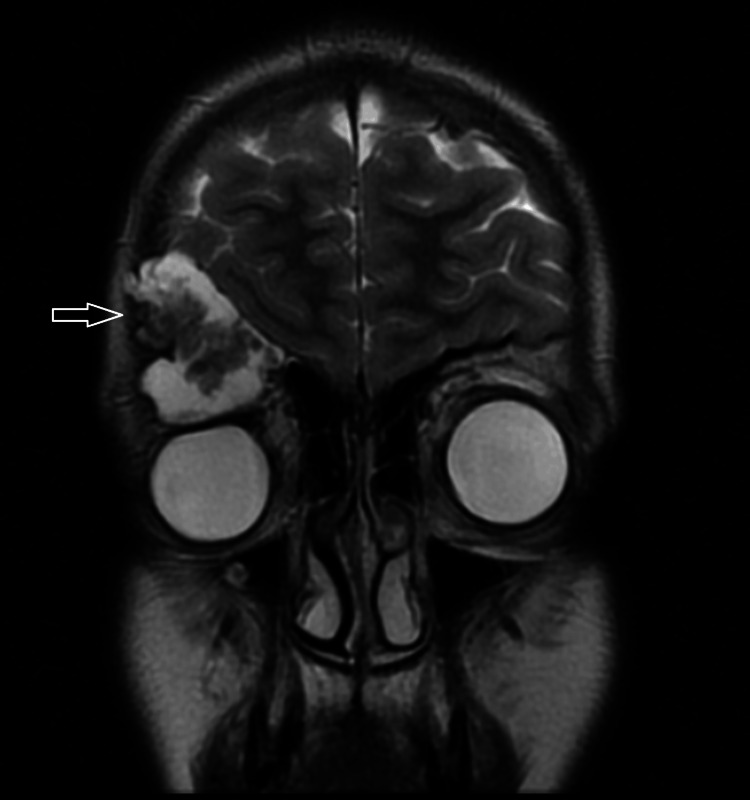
T2-weighted image shows right supero-lateral intraorbital 2.0x1.0x0.5 cm osseous expansile lobulated cystic lesion with internal soft tissue component exerting mass effect over the right globe downward as well as thinning of the superior orbital bone without intracranial or intraocular component.
